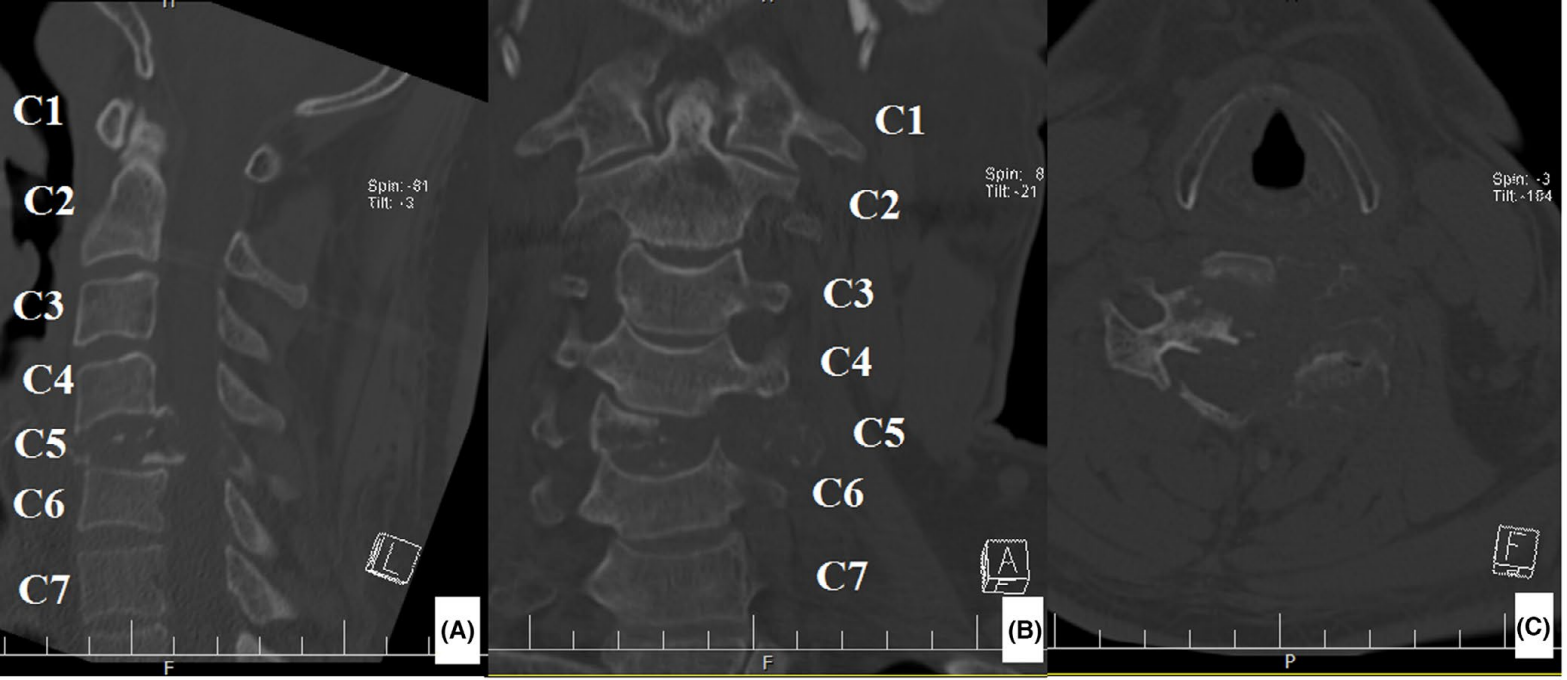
Preoperative cervical CT scan reveals a C5 lytic lesion affecting vertebral body, left sided lamina and pedicle
